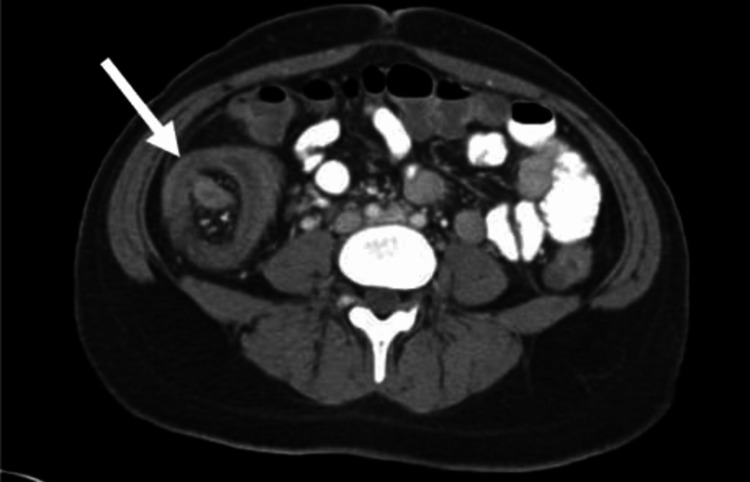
Computed tomography scan imaging.
